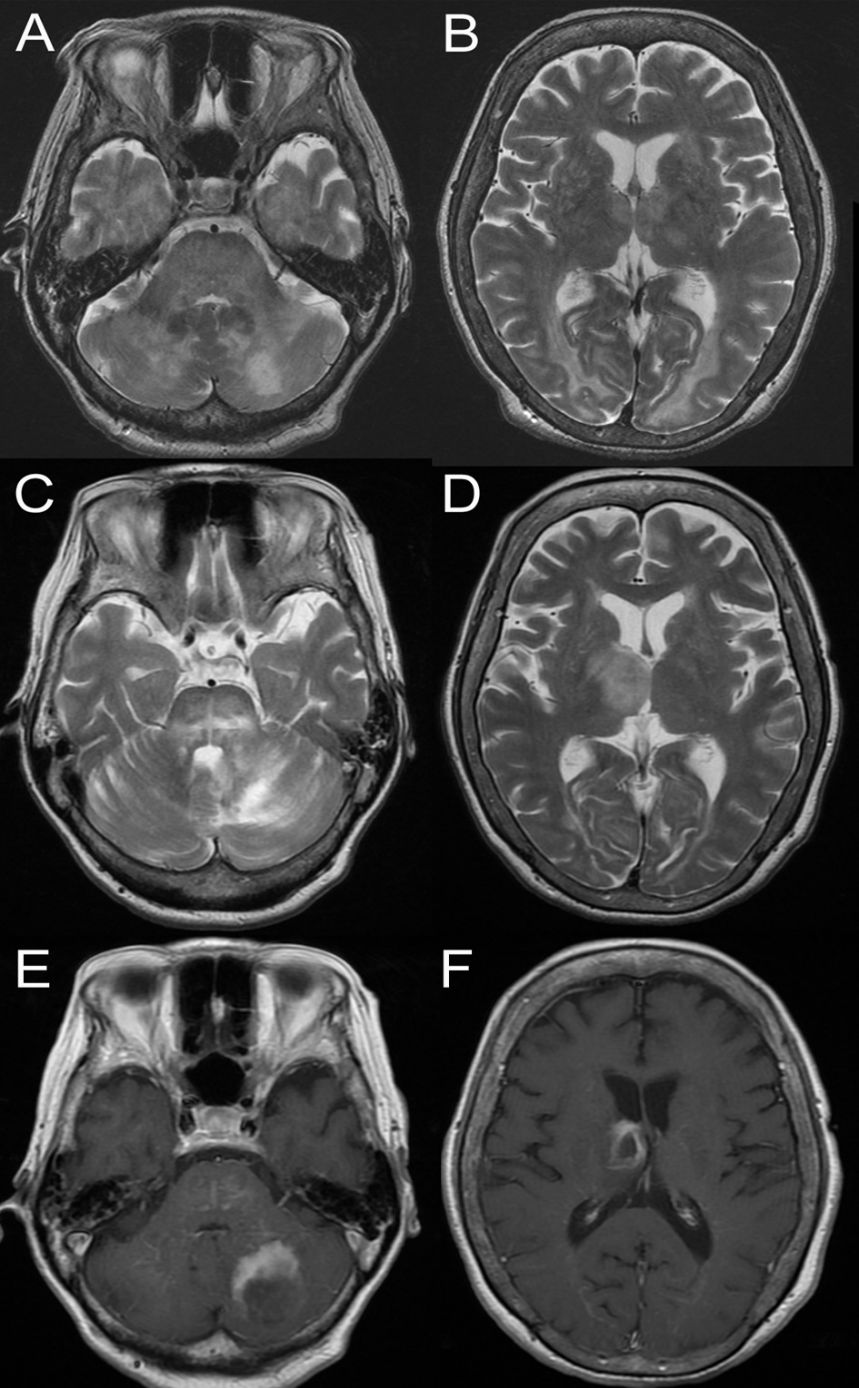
Brain magnetic resonance imaging of the patient. A-B, T2-weighed imaging at initial presentation: (A) Hyperintense signal in the bilateral cerebellar hemispheres; (B) Hyperintensity noted in the bilateral thalamus and bioccipital subcortex; C-F, T2-weighted imaging and T1-weighted imaging with gadolinium enhancement immediately before brain biopsy; (C) Hyperintense lesions noted in the pons and the left side of the cerebellum; (D) Progression of the lesion of the thalamus on the right; (E) Ring-enhancing lesion of the cerebellum; (F) Ring-enhancing lesion of the thalamus.
